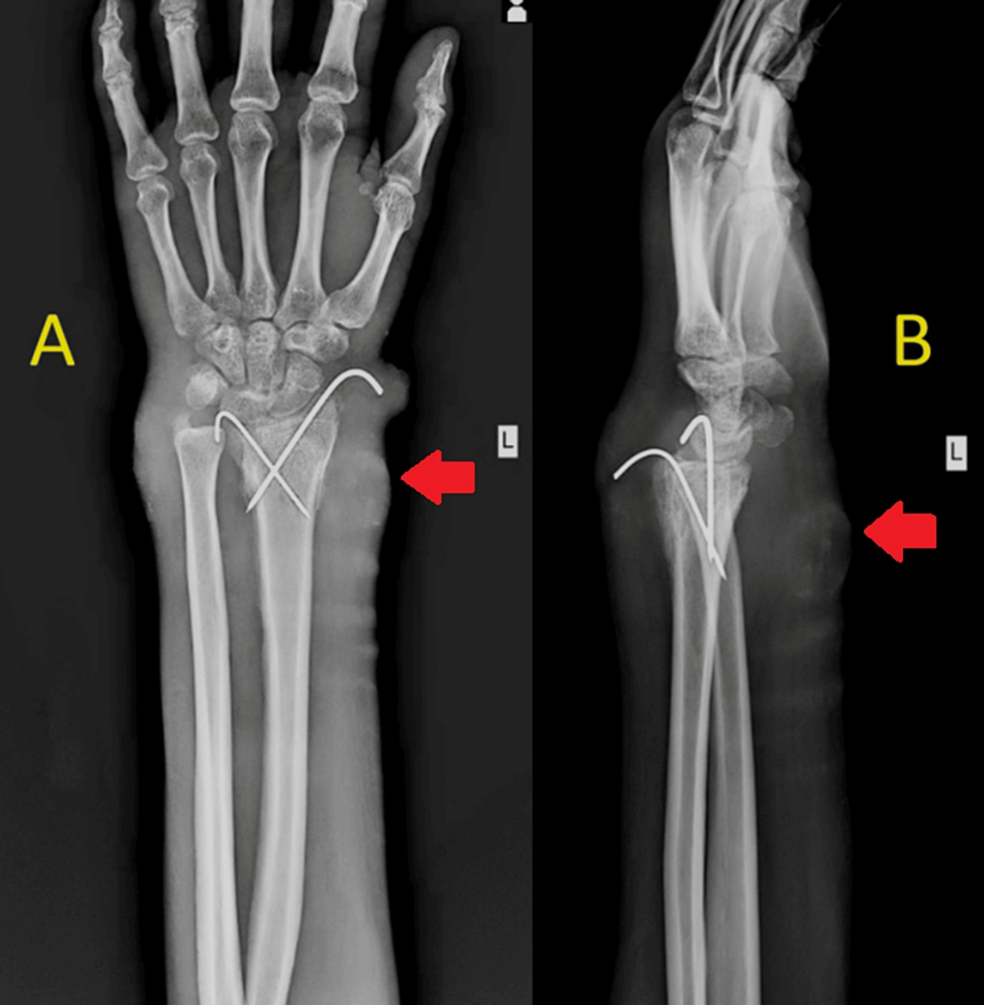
X-rays of left wrist anteroposterior (A) and lateral (B) views after six months showing the enlarged soft tissue shadow and sinus formation over the volar and dorsal aspect with callous seen along the medial, lateral anterior, and posterior cortex (red arrows) with two K-wires in situ.
